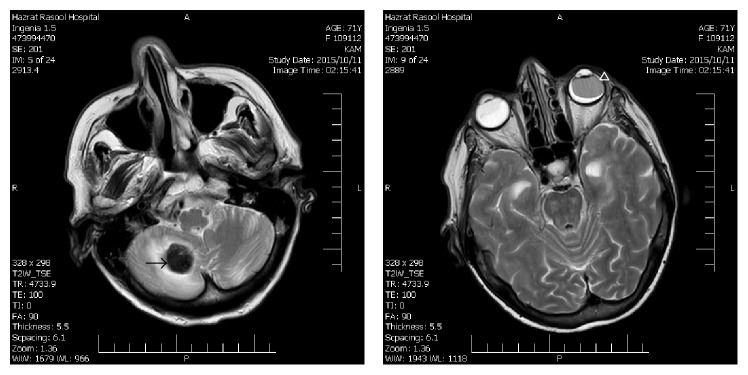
T2-weighted MR images. The intraocular silicone oil (arrowhead) is hypointense relative to the vitreous of the fellow eye and hyperintense relative to the cerebellar hemorrhage (arrow). Extensive retinal detachment is evident in the right eye.
